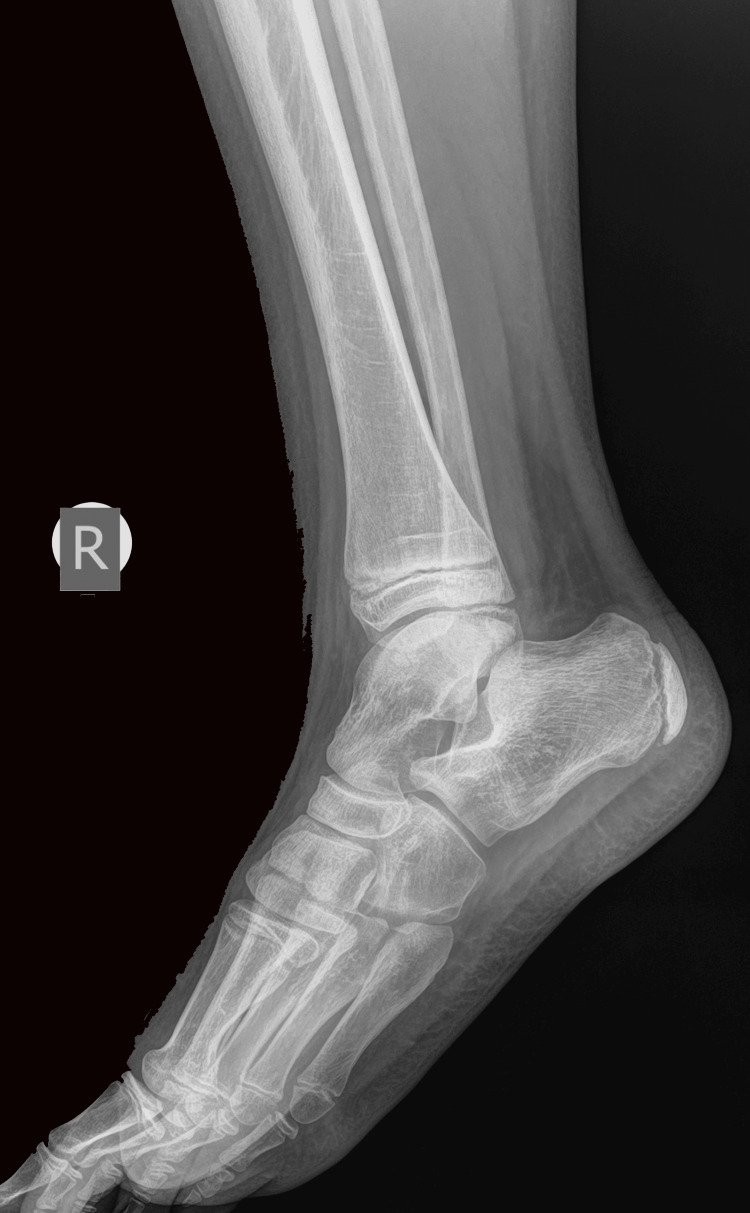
Lateral X-ray of the right ankle
